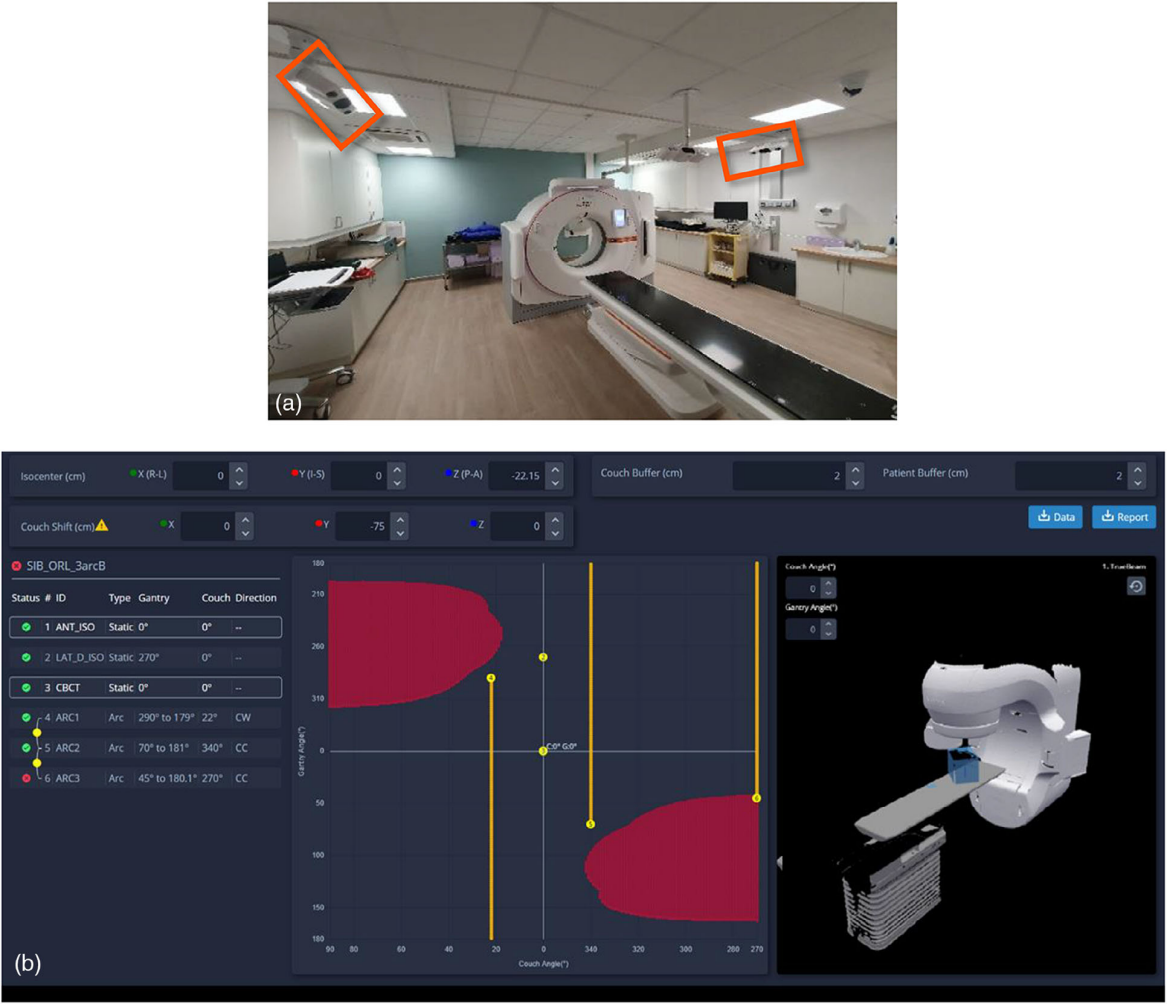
Camera setup of the MapRT system on the local Siemens Go.Sim CT (a), overview of the MapRT software interface showcasing the 3D model of the treatment room (right), the clearance map with collision areas in red and arcs/static beams in yellow (center), and collision checks results for each treatment field and for the transition between fields (left) (b).
